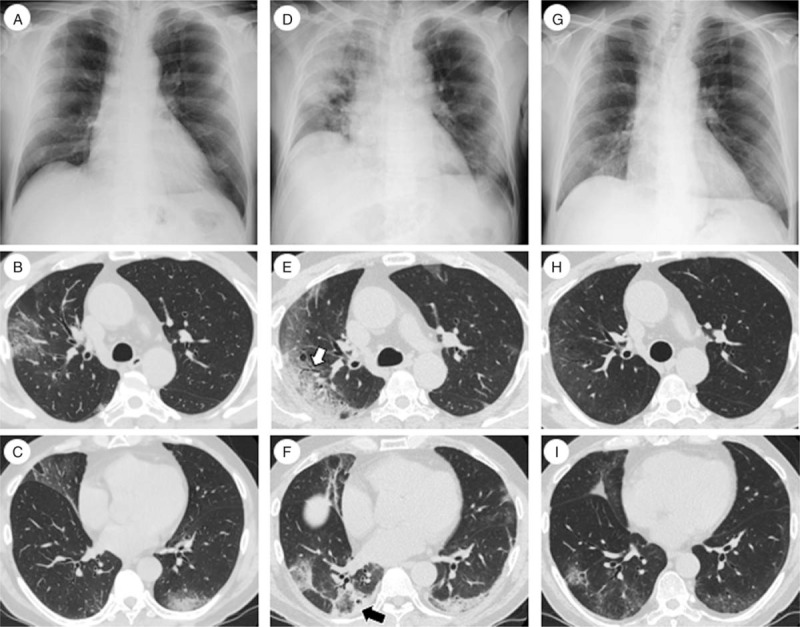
Chest imaging of Case 1. (A) Chest X-ray on admission. A slight ground-glass opacity (GGO) was observed in the right lung. (B, C) Chest computed tomography (CT) on admission revealed bilateral patchy GGO that was predominantly distributed throughout subpleural areas. (D) Chest X-ray on day 6 post hospital admission (taken immediately before corticosteroid administration) revealed consolidations and marked lung volume loss. (E, F) Chest CT on day 6. Consolidations developed bilaterally, some with band-like shapes. A central GGO surrounded by ring-shaped areas of consolidation (reversed halo sign) was observed (black arrow). Some GGOs were accompanied by traction bronchiectasis (white arrow). The volume of both lower lobes was decreased. (G) Chest X-ray and (H, I) Chest CT on day 23 revealed improvement of lung infiltration and recovery of lung volume. CT = computed tomography.
